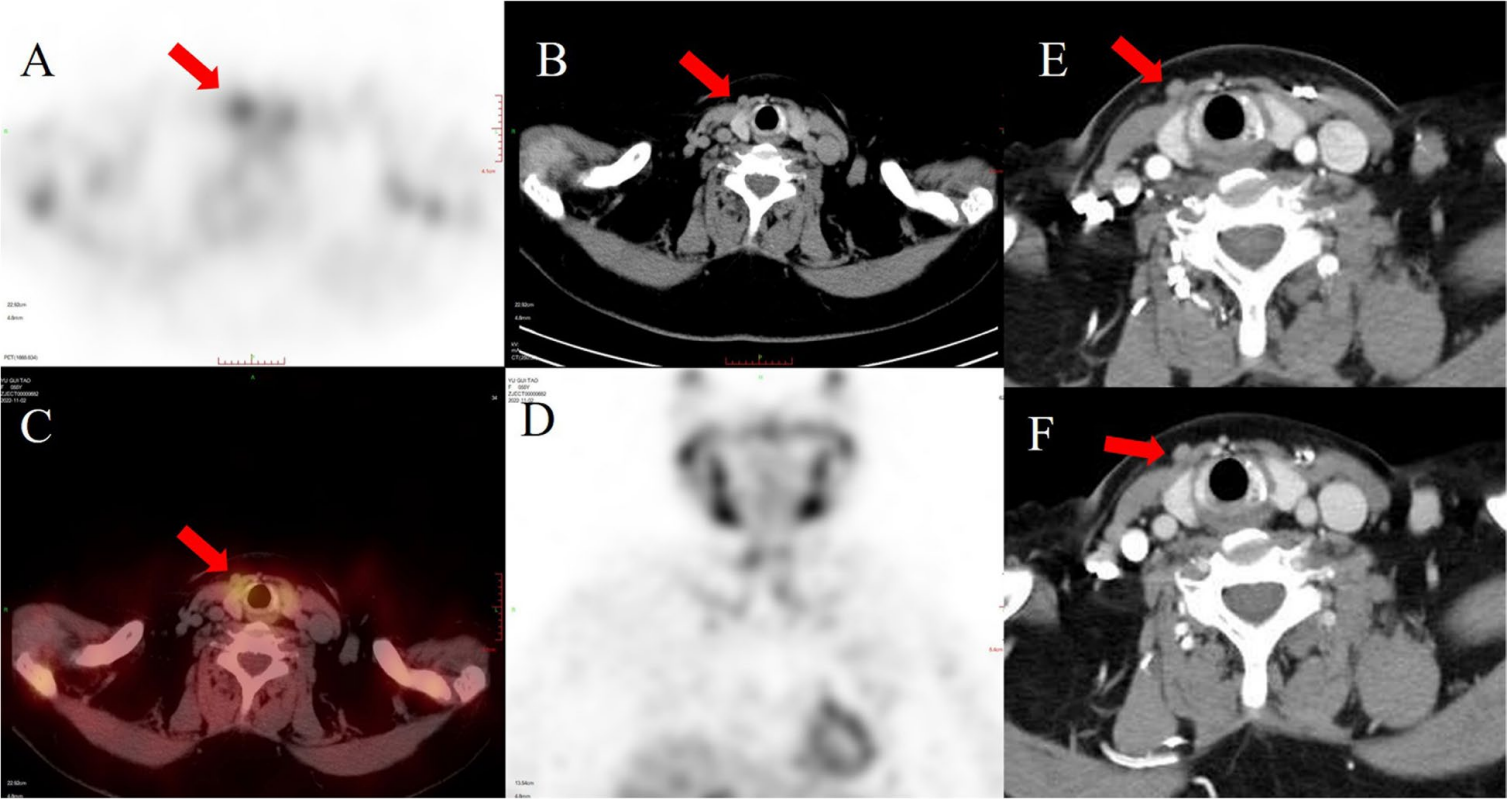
The 99mTc-MIBI SPECT/CT scan also shows a mild focus tracer accumulation with a low-density nodule located subcutaneously in the anterior neck (red arrows: A SPECT, B axial image, C axial SPECT/CT fusion image, D coronal SPECT image). Contrast-enhanced CT scan reveals arterial-phase (D) and delayed-phase (E) axial images of mildly enhanced nodules (red arrows) located subcutaneously in the anterior neck
